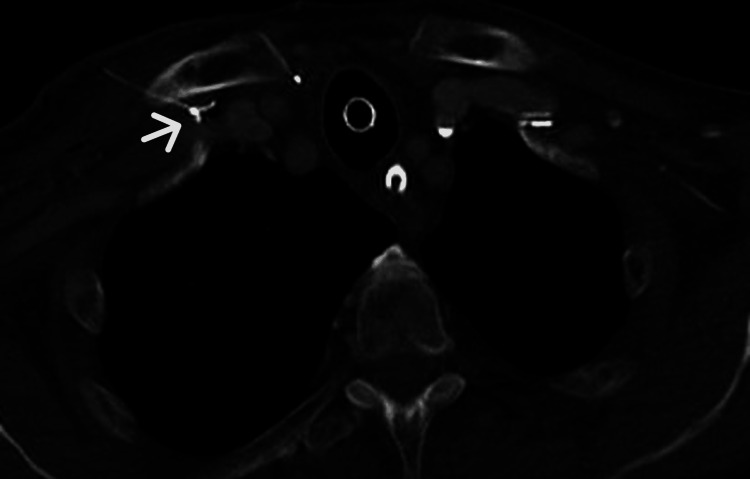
Axial contrast-enhanced CT scan Axial contrast-enhanced CT scan showing the guidewire compressed within the costoclavicular space. The arrow indicates the guidewire trapped between the clavicle and the first rib, providing direct and definitive evidence of mechanical entrapment.
